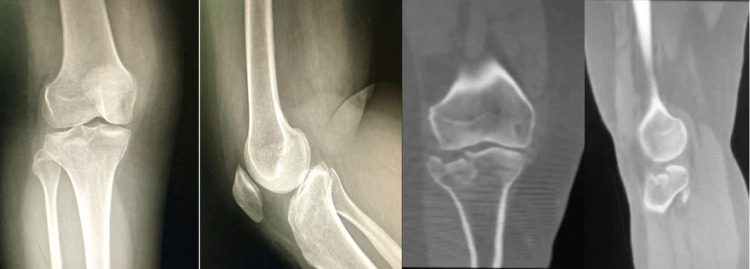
Anteroposterior and lateral X-ray along with coronal and sagittal CT scan of the knee showing a depressed lateral plateau fracture with significant displacement (Schatzker Type III tibial plateau fracture).
